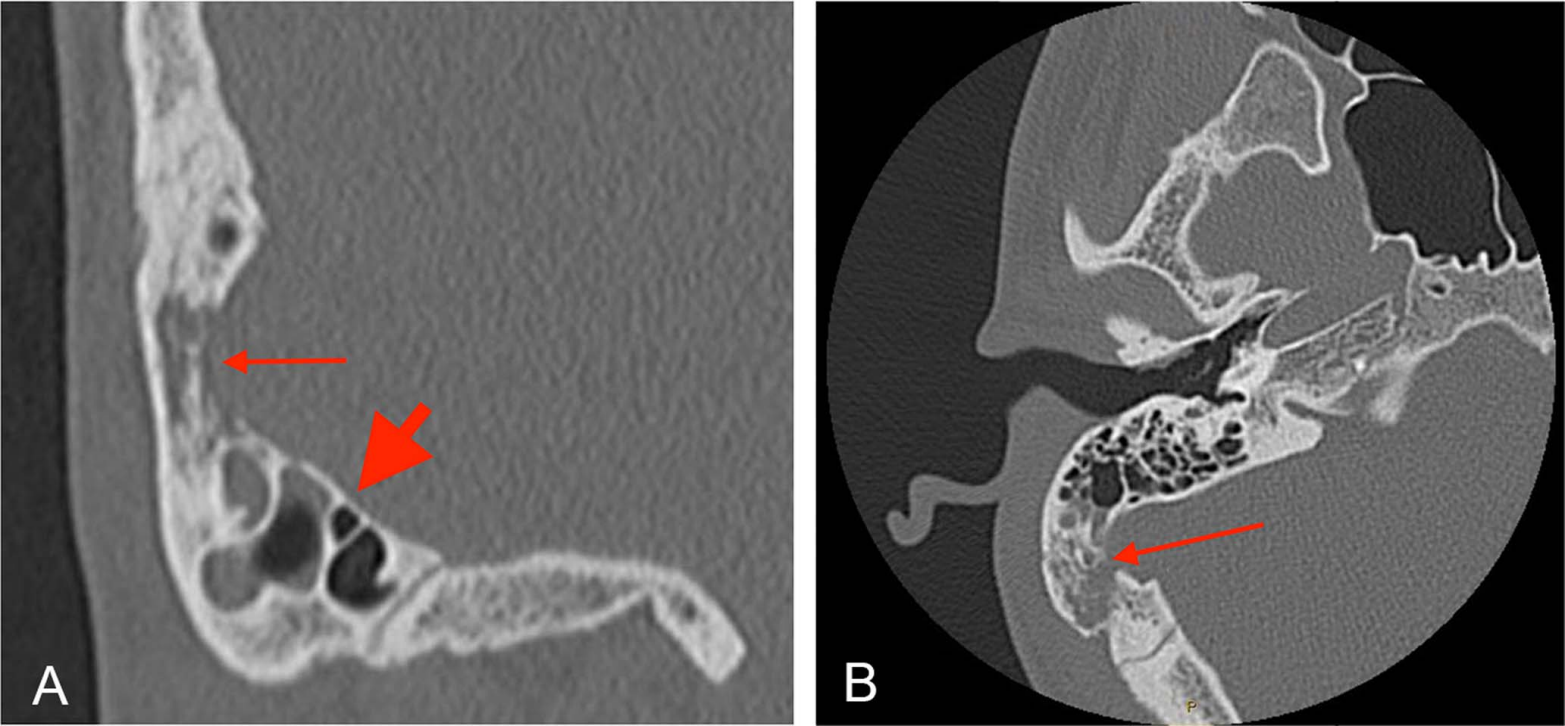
Initial coronal (A) and axial (B) non–contrast-enhanced computed tomography scans of the right temporal bone show patchy opacification of the posterior right mastoid air cells with erosion of the septa and overlying mastoid cortex. In view A, the thin arrow indicates erosion of bone, and the thick arrow indicates opacification of the mastoid air cells. In view B, the thin arrow indicates erosion of bone.
